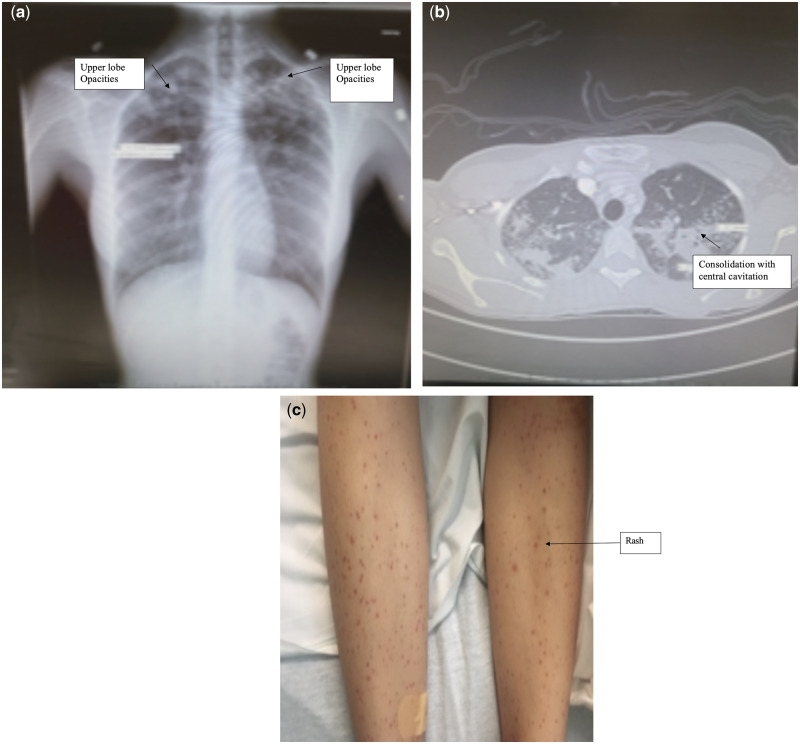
Chest XRAY showing bilateral upper lobe opacities
